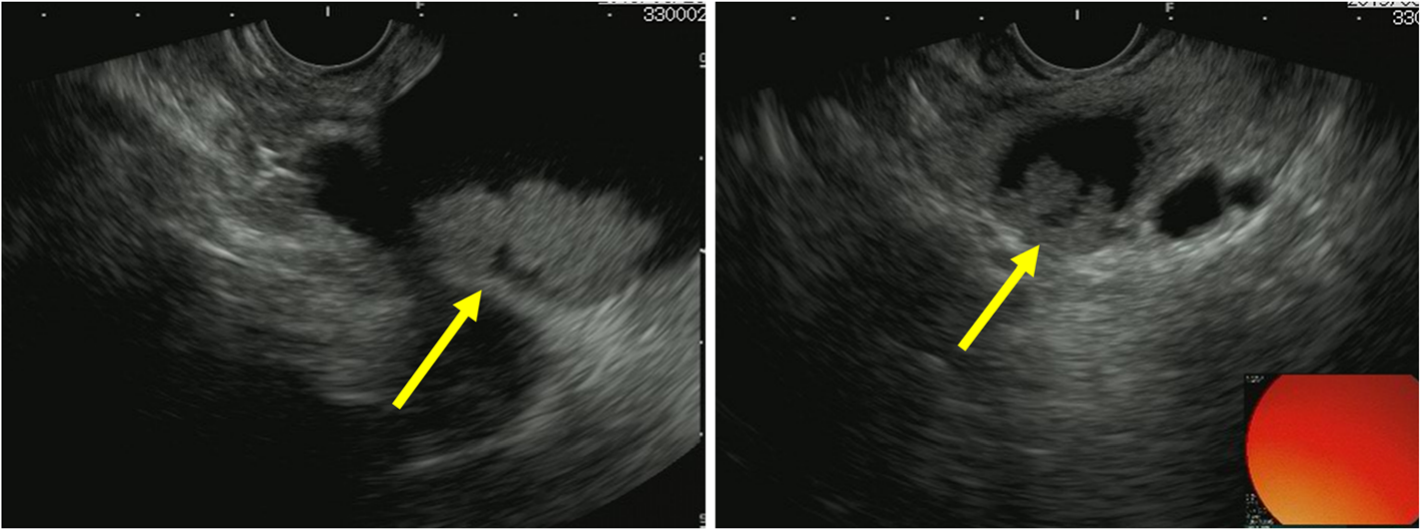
Endoscopic ultrasonography (EUS) imaging findings. EUS revealed that the cystic tumor of the pancreatic head was a 35-mm solitary cyst with a 24-mm mural nodule, and the cystic tumor of the pancreatic tail was a 20-mm solitary cyst with a mural nodule
